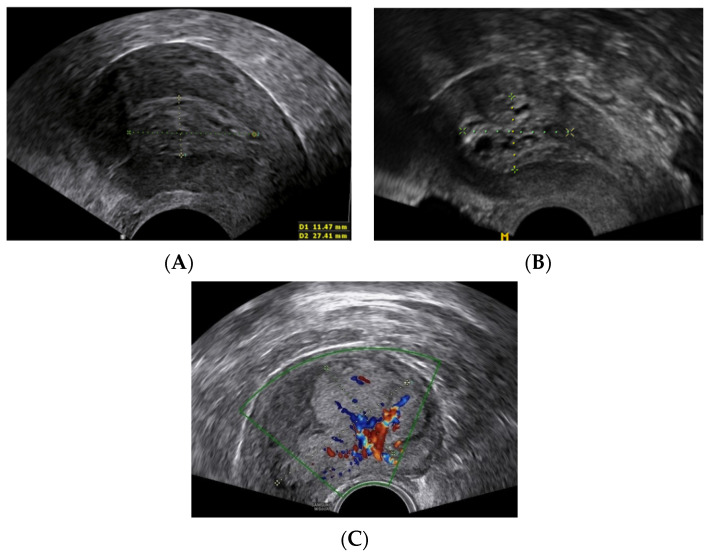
Tumor ultrasound characteristics. (A) No myometrial invasion; (B) myometrial invasion <½; (C) myometrial invasion >½.
